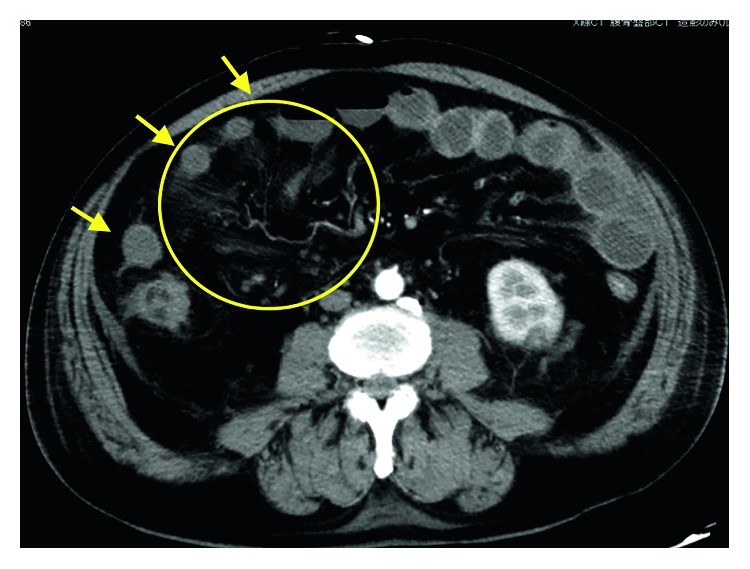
Axial-section contrast-enhanced CT of the abdomen performed in the ICU. Poor contrast effects can be seen in the small intestine (arrows). Disruption of peripheral blood vessels of the superior mesenteric artery is apparent (circled).
